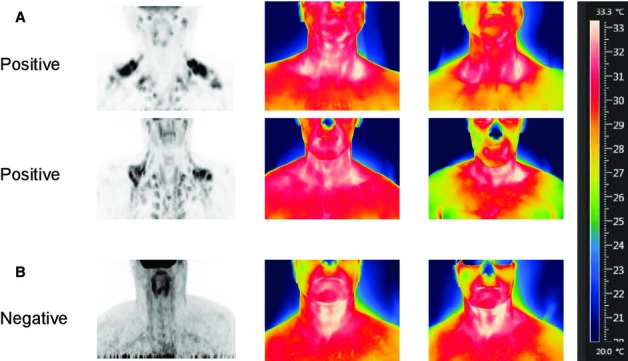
Representative PET‐CT and thermography images before and after 120‐min cooling for (A) two BAT‐positive subjects and (B) one BAT‐negative subject. In A, the thermograms for both PET‐positive subjects indicate that the skin temperatures in the SCVs are higher than the surrounding areas and with cooling, there is a fall in chest temperature as denoted by the color change. (B) Shows minimal difference in temperature between SCV and control chest areas. With cooling, there is no fall in chest temperature.
